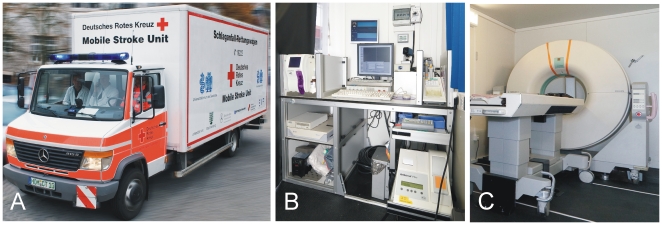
Mobile Stroke Unit. An ambulance (A) equipped with point-of care laboratory system and telemedicine devices (B) and CT (C) required for prehospital stroke treatment.
